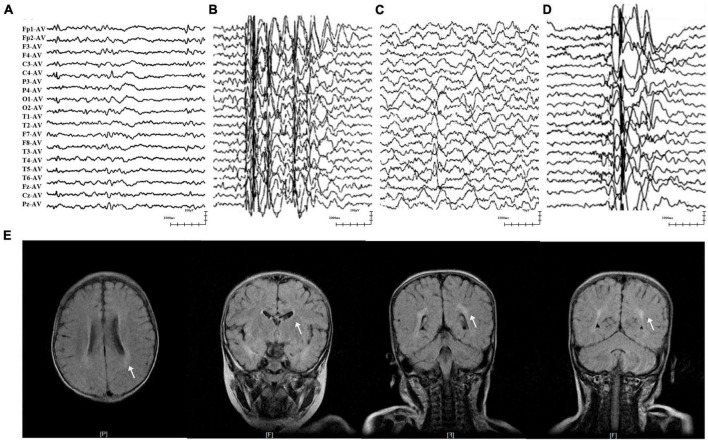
Representative EEG and MRI of patients with *GABRA1* variants. (A) Interictal EEG of case 1 showed multifocal waves at the age of 3 years. (B) Interictal EEG of case 2 showed generalized spike-slow waves at the age of 10 years. (C) Interictal EEG of case 4 showed right temporal slow waves at the age of 3.5 years. (D) Interictal EEG of case 5 showed generalized spike-slow waves and irregular 0.5–3 slow activity bursts 0.5–2 s during sleep. (E) The MRI of case 5 showed an abnormal white matter signal around the posterior horn of the lateral ventricle.
